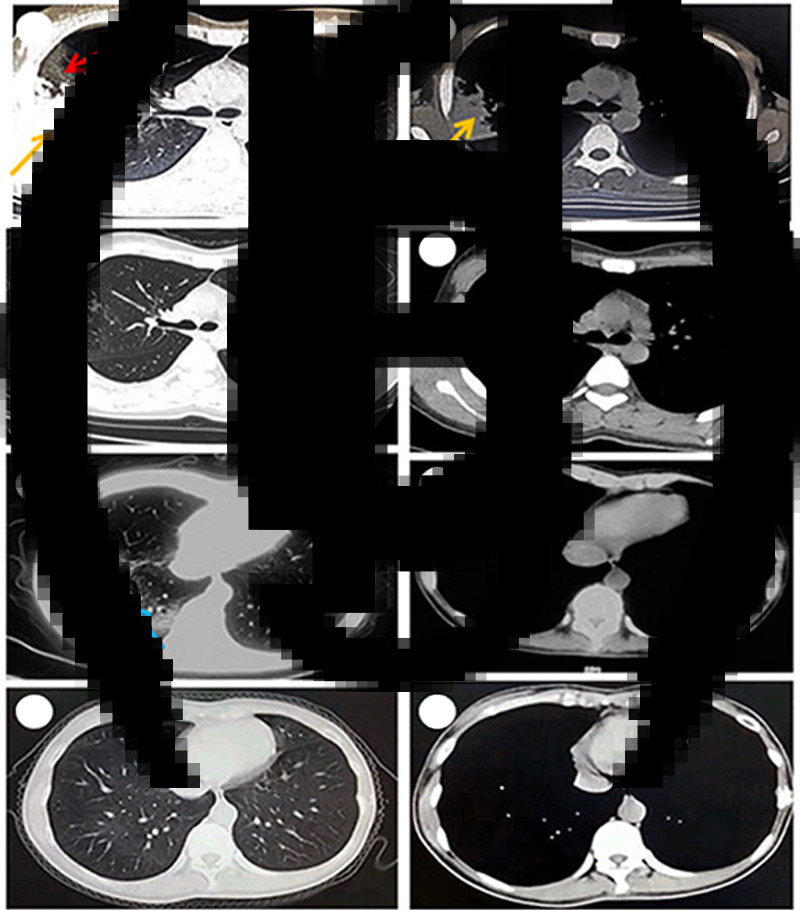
Chest CT of case 1 obtained on 18 February 2020 showing ground glass opacity (a, red arrow) and consolidation (a, b, yellow arrow) in right upper lobe of lung. Chest CT of case 1 obtained on 2 March 2020 showing significant improvement after 1 week' treatment (c, d). Chest CT of case 2 obtained on 10 February 2020 showing ground glass opacity in right lower of lung (e, blue arrow). Chest CT of case 2 obtained on 23 February 2020 showing normal after 10 days of treatment (g, h).
